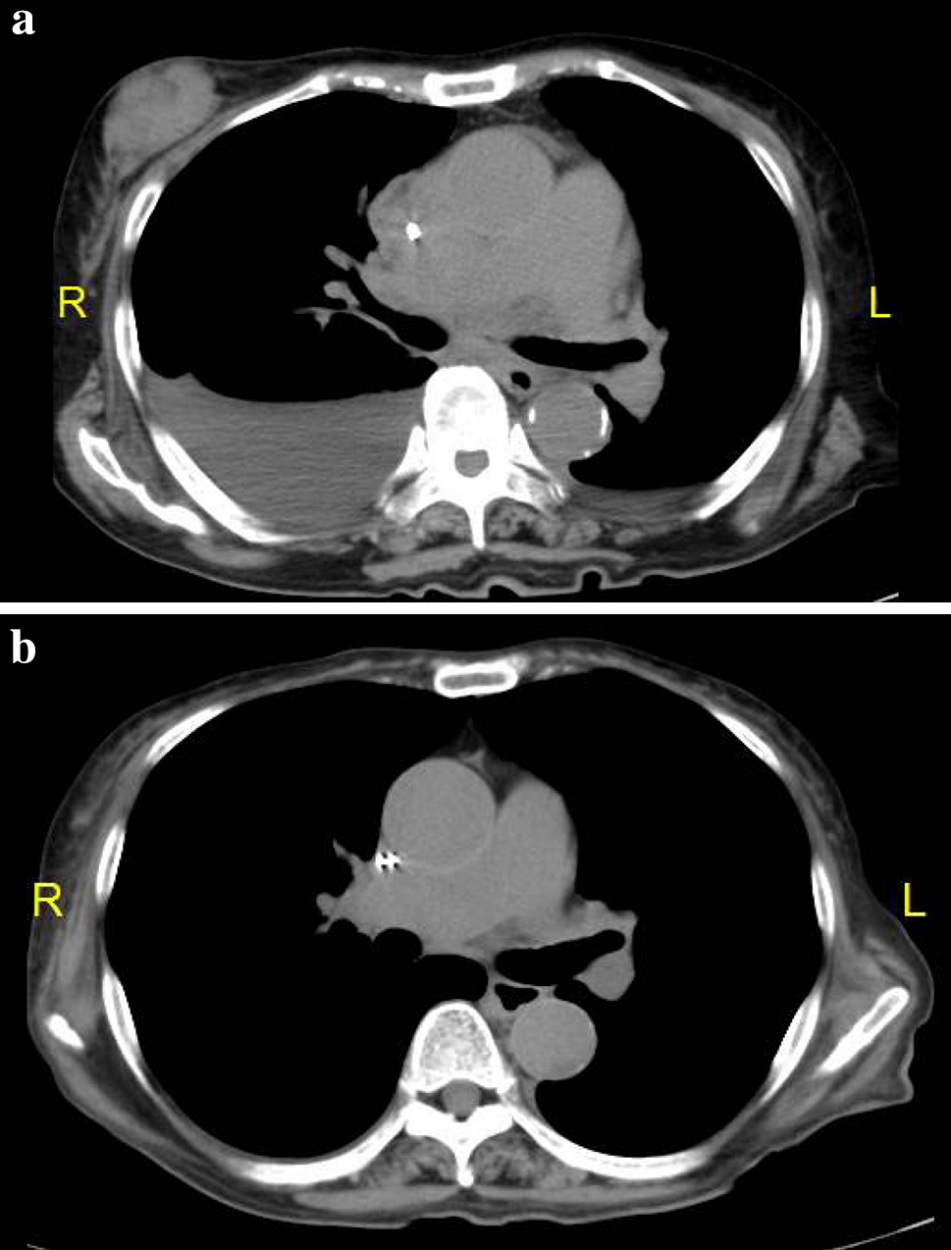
a Computed tomography showed a round mass, 4.8 × 2.6 × 5 cm, in the outer *upper* quadrant of the *right* breast with pleural effusions in both lungs before treatment. b The mass in the *right* breast and bilateral pleural effusions disappeared with nearly complete response after 6 cycles of CHOP chemotherapy
